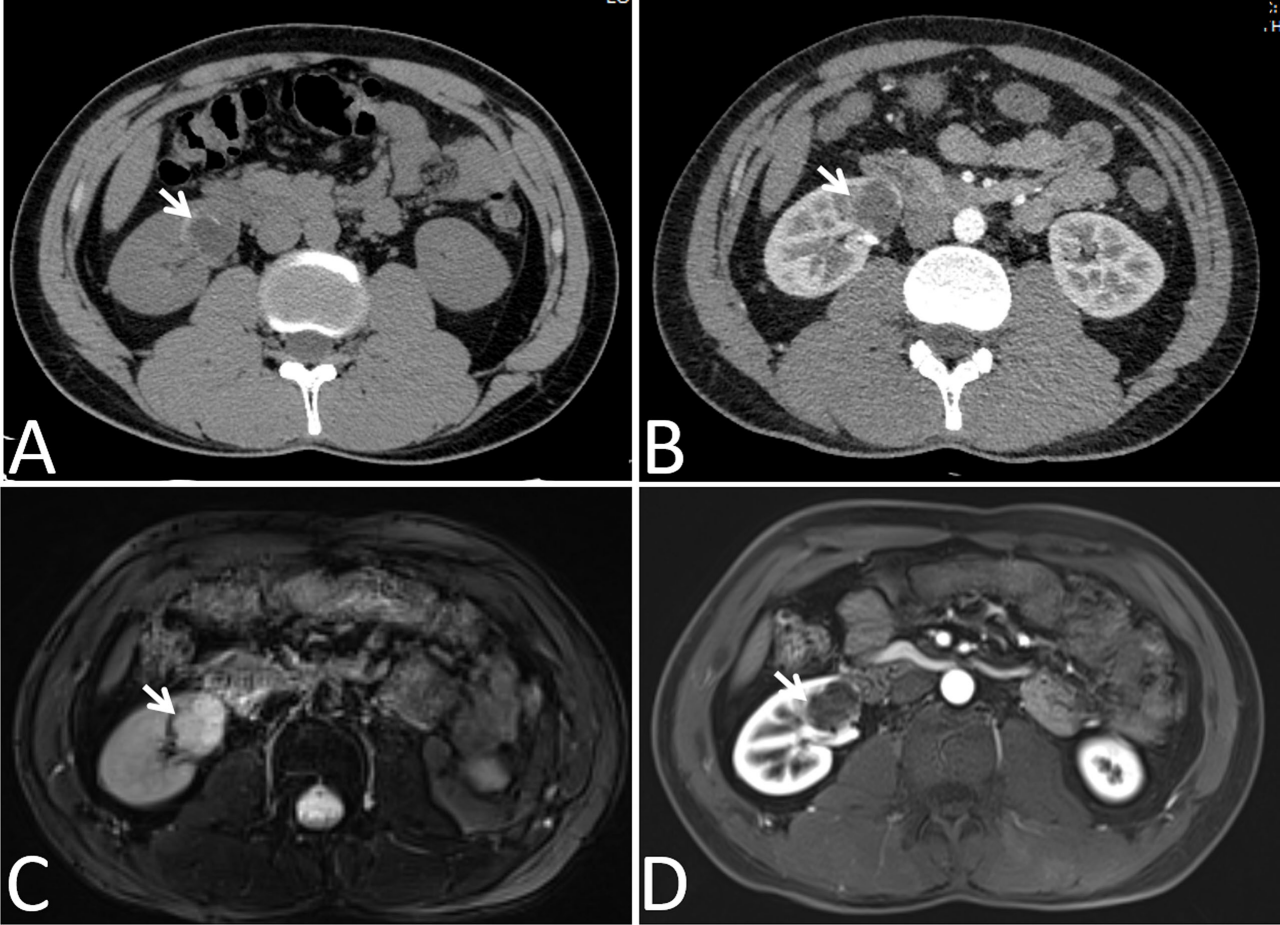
Images in a 24-year-old man had a cyst with calcium deposits in the right kidney confirmed by pathology. (A) Axial non-contrast-enhanced CT image shown a cystic mass with thick crescent calcification (arrow) in its wall, (B) Axial contrast-enhanced CT image shown the wall of cyst was obscured by the high density calcification (arrow), (C) Axial T2-weighted MR image shown irregular thickening of the lesion wall (arrows), (D) Axial gadolinium-enhanced fat-suppressed T1-weighted MR image shown no measurable enhancement of the wall (arrows). Calcification depicted on the CT image was not mistaken for enhancement on the MR image.
